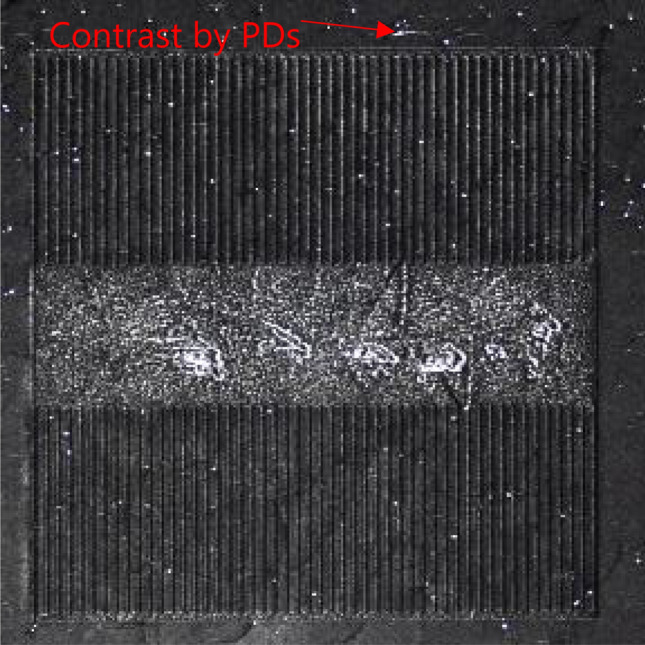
X-ray topographic image of the PiN diode shown in Fig. 3a.
